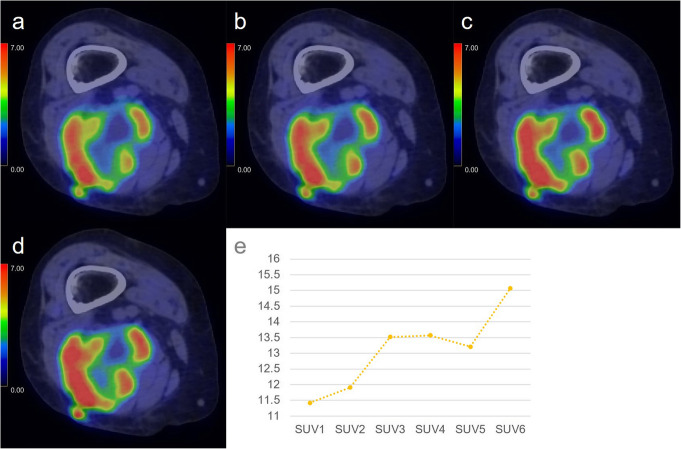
An 85-year-old woman with diffuse large B cell lymphoma in the right thigh. Dynamic positron-emission tomography/computed tomography (PET/CT) (a–d) images are shown. In all dynamic phases (a–d), methionine accumulation in the soft tissue tumour is inhomogeneous, and intense accumulation is identifiable in the peripheral regions from the dynamic first phase (SUVmax: dynamic first phase (a), 11.42; dynamic second phase (b), 11.91; dynamic third phase, 13.52; dynamic fourth phase (c), 13.57; dynamic fifth phase, 13.21; dynamic sixth phase (d), 15.07). The accumulation gradually becomes more intense in most parts of the tumour during the dynamic phases (a–d). The time-SUVmax curve (e) shows a high level in SUVmax at the dynamic first phase (5–15 min after injection) and a gradual increase during the dynamic phases
